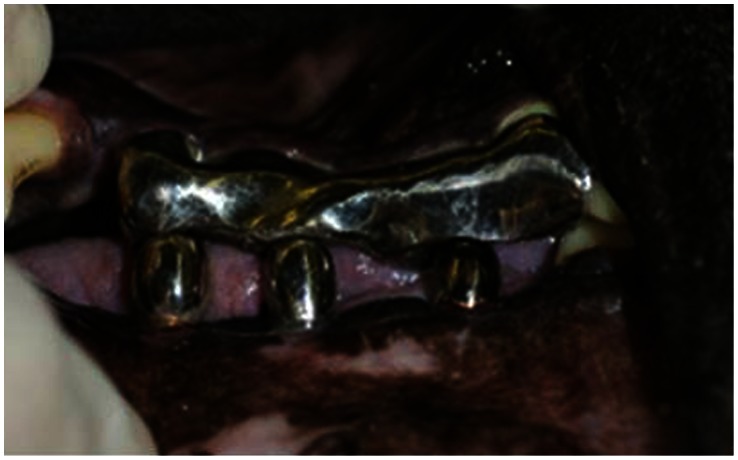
Measurement of vertical marginal bone loss around implant (MBL). (1) IR: Implant length of Radiograph (perpendicular distance from the implant shoulder to the most apical aspect of the implant.) and (2) MBLR: Radiographically evaluated MBL. MBLRm is the average perpendicular distance from implant shoulder to first visible apical bone-to-implant contact at the mesial side. MBLRd is the same at the distal side. MBLR is calculated by the average value of MBLRm and MBLRd.
